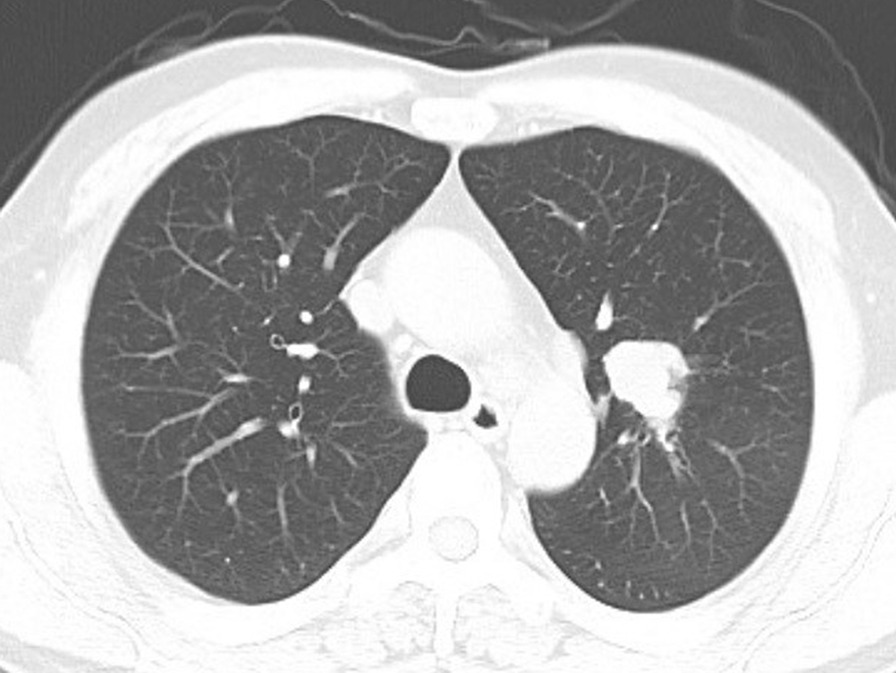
Imaging characteristics of peripheral pLCNEC. Chest CT showed a lobulated mass with a clear boundary and obvious short burrs
